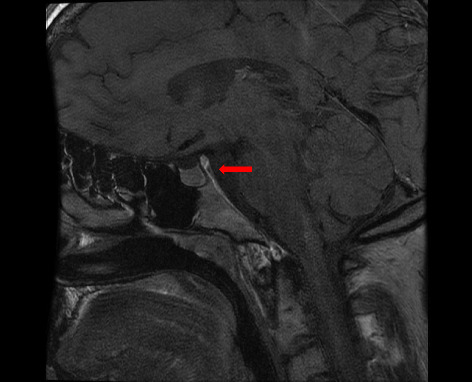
Brain magnetic resonance imaging (MRI). Sagittal view shows absence of normal hyperintense T1 signal in the neurohypophysis in the posterior intrasellar localization (arrow) in relation to diabetes insipidus.
